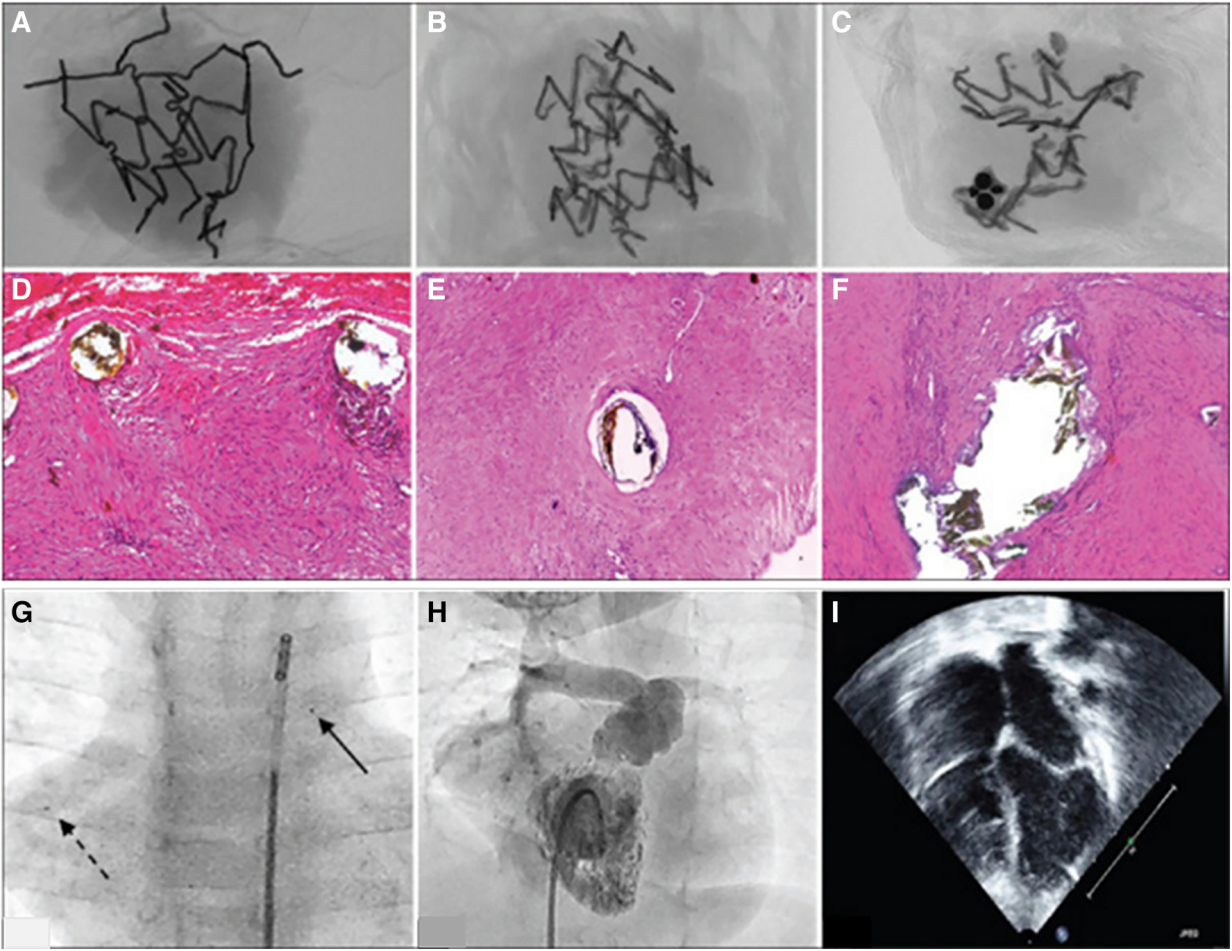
Figure adapted with permission from reference (73). (A–C) Show micro CT scans from stent materials explanted from three patients at 6 months (A) and 16 months (A,C). At 6 months, macrophages with hemosiderin in the cells are seen near the strut (D) At 16 months, the authors report the tissue repair was resolved without necrocytosis (E,F). (G) Shows embolized stent fragments to the right pulmonary artery (broken arrow) compared to the original implanted stent (solid arrow). (H) Right ventricular angiogram showing that flow is not compromised where the stent material was embolized. (I) Shows that the right atrium is not enlarged and there is no more septal bulge toward the left atrium, post right ventricle overhaul and right ventricular outflow tract reconstruction.
